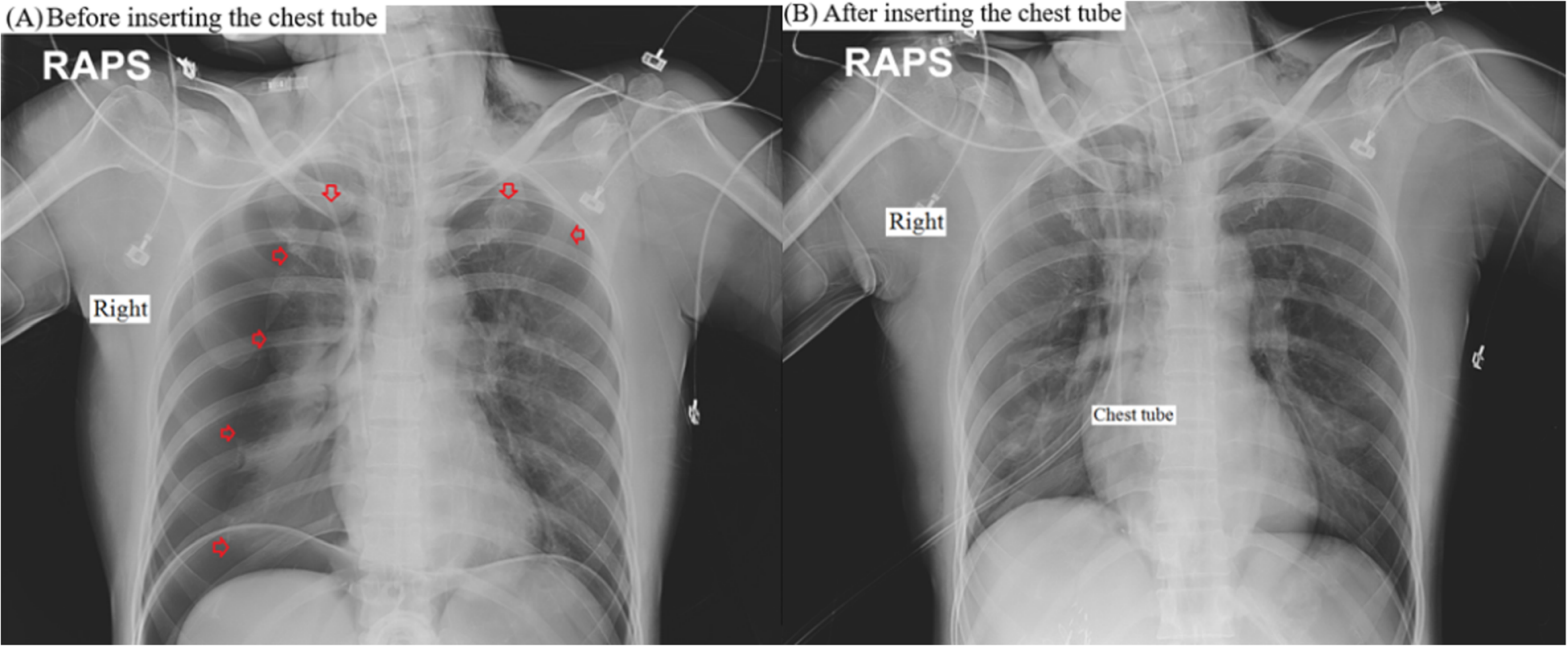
Chest radiography. a Before inserting the chest tube. b After inserting the chest tube. Arrows indicate the visceral pleural line with the absence of vascular marking beyond the pleural line
